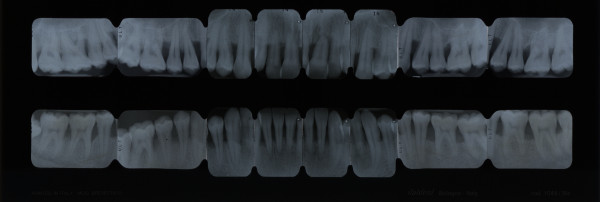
Full-mouth series periapical X-rays show diffuse horizontal bone loss.
